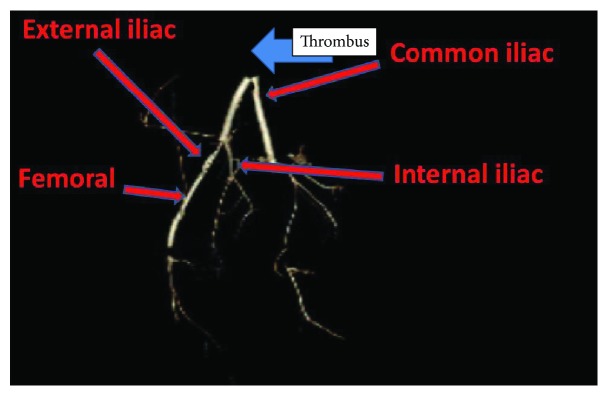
CT angiogram reconstruct showing large segmental aortic occlusions.
